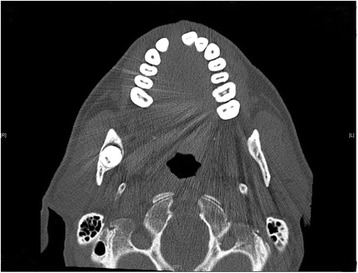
Axial cone beam scan showing the impacted tooth in the right sigmoid notch
